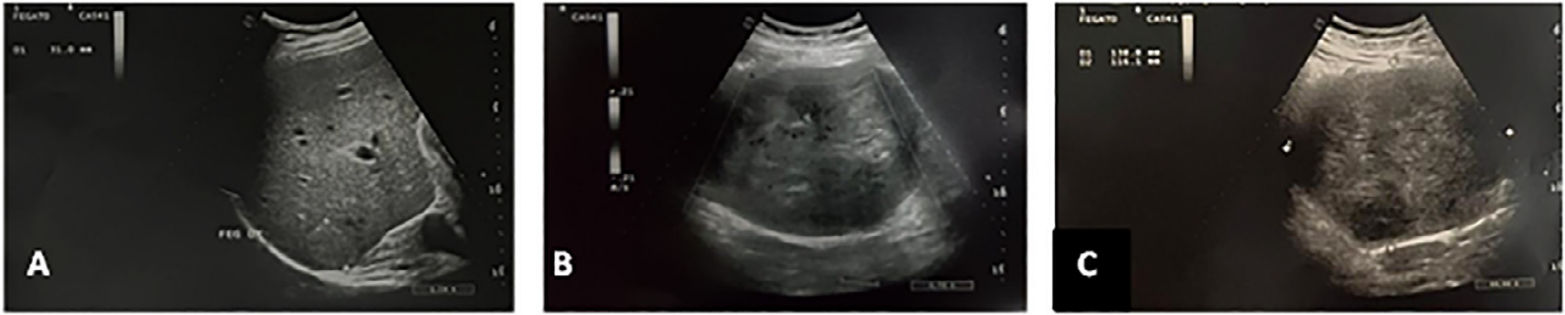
Angioma of the VI-VIIth segment: a nodukar formation of 3 mm, hyperechoic and with clear margins (A). Voluminous solid nodular formation of an expansive character measuring 14 × 11.6 cm whose echoctructure was heterogenous (C), and with a significant vascularization at Doppler (B).
